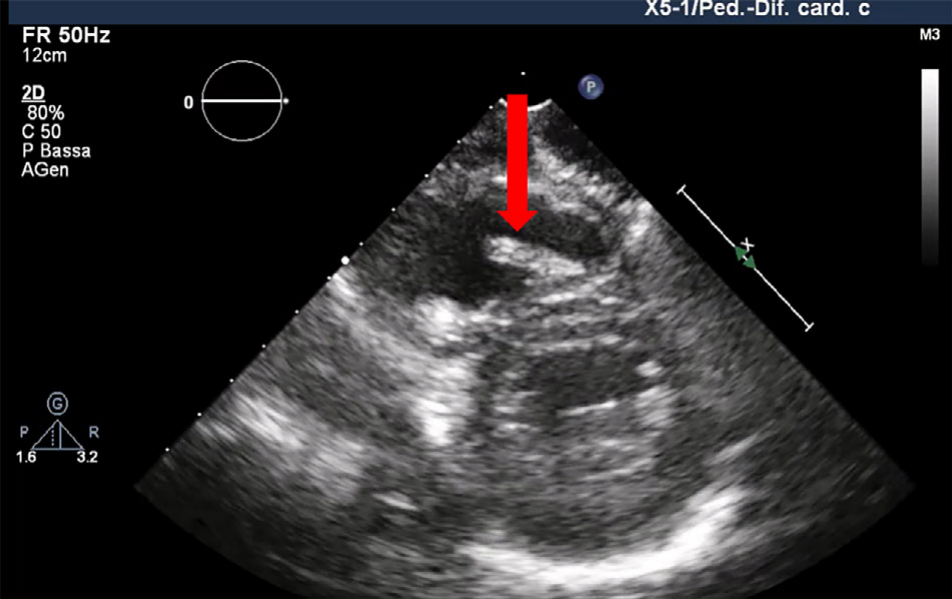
Transthoracic echocardiography showing the leadless pacemaker correctly positioned on the mid‐interventricular septum (red arrow). [Colour figure can be viewed at wileyonlinelibrary.com]
